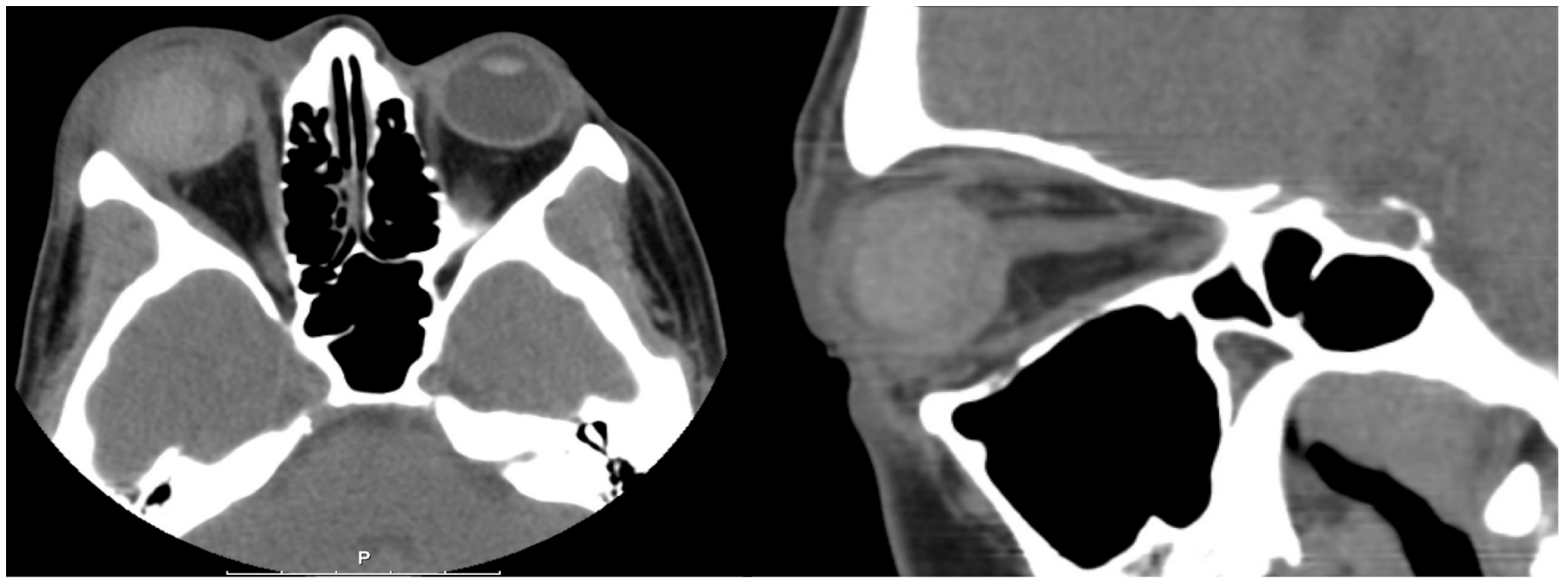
29 year old woman presenting with loss of eye sight and ocular pain. Non-contrast enhanced CT revealed an anterior bulbus perforation with hemorrhage in the anterior chamber and the vitreous humor. No pathological changes retrobulbar and no foreign body was noted.
